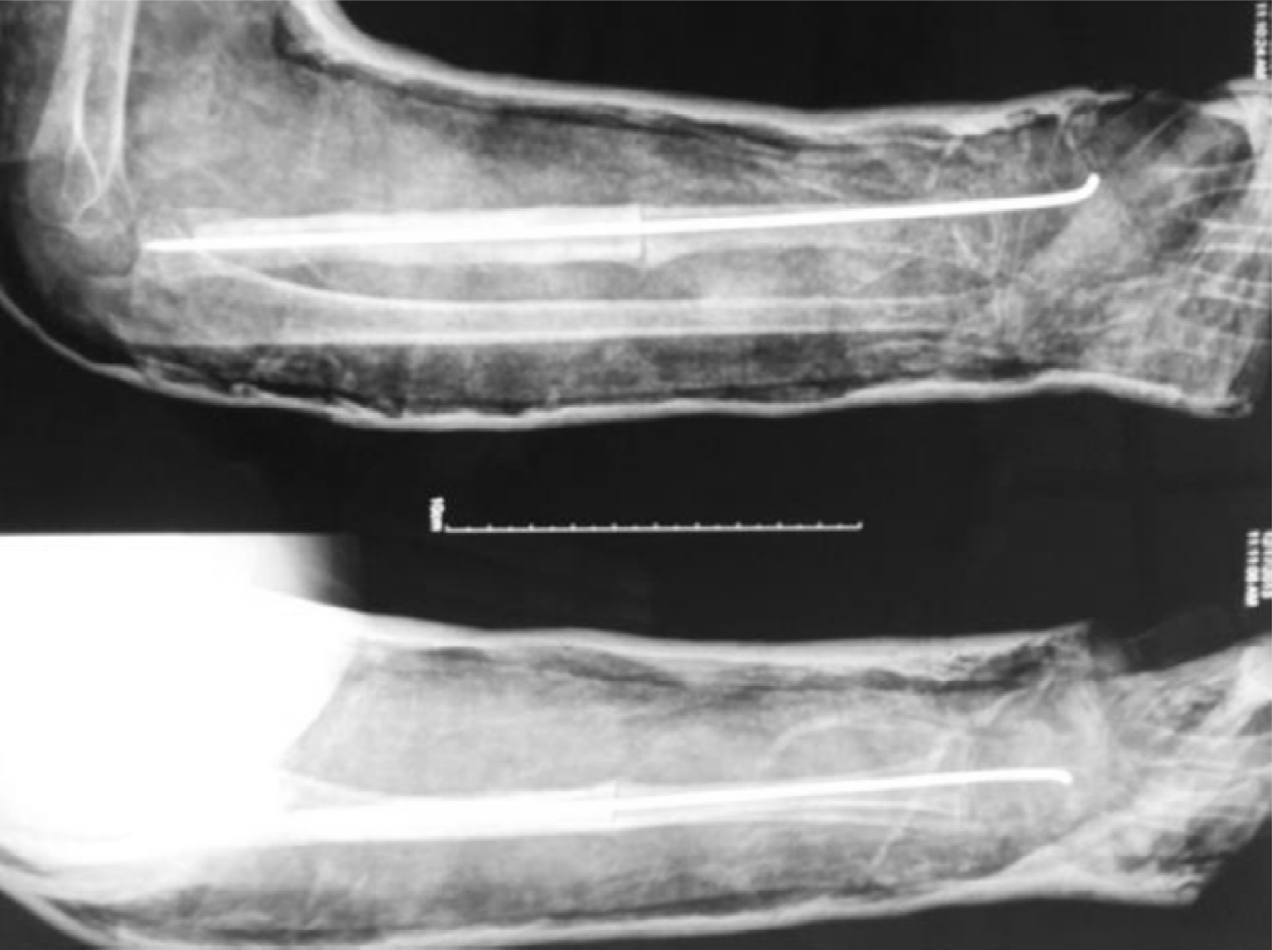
Postoperative X-ray showing reconstruction of the radius using maternal fibular graft fixed with an intramedullary K-wire.
